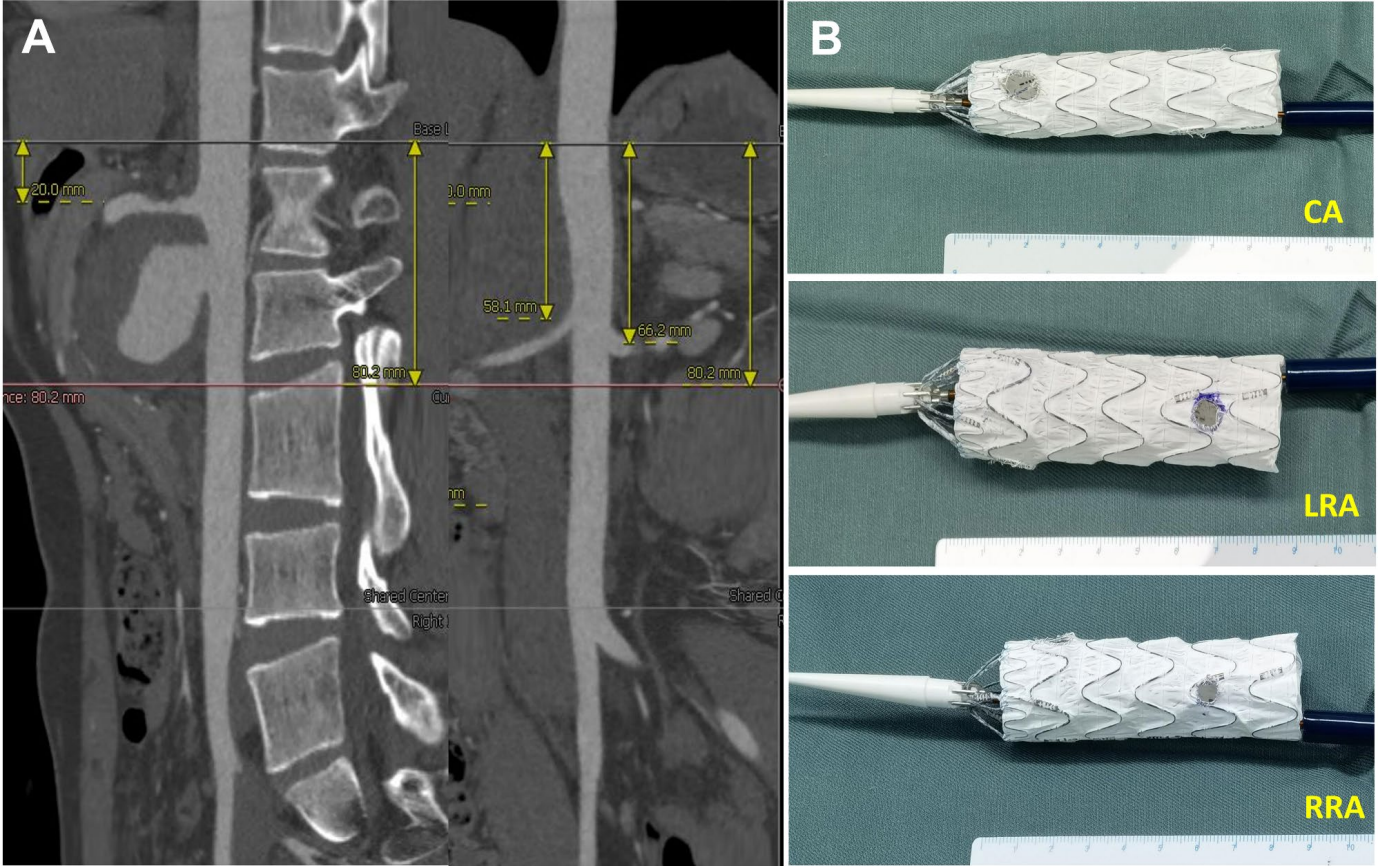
Physician-modified three-fenestration endograft was created based on centre lumen line reconstruction of the preoperative CTA. (A) centre lumen line reconstruction. (B) three fenestrations were created at planned positions for the celiac trunk (CT), left renal artery (LRA) and right renal artery (RRA).
